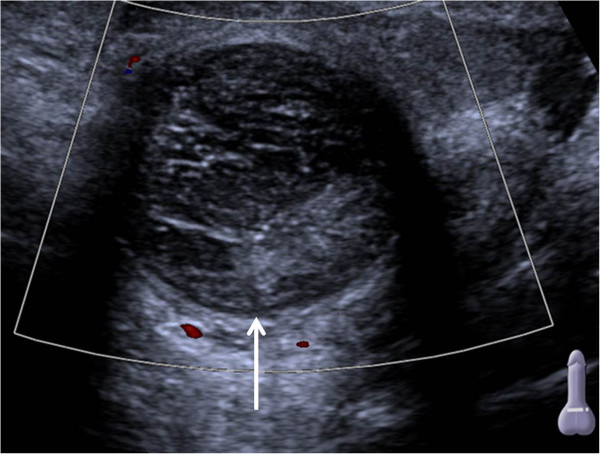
Color Doppler ultrasound (transversal axis) of the proximal penis. Arrow shows absence of flow in the thrombus.
